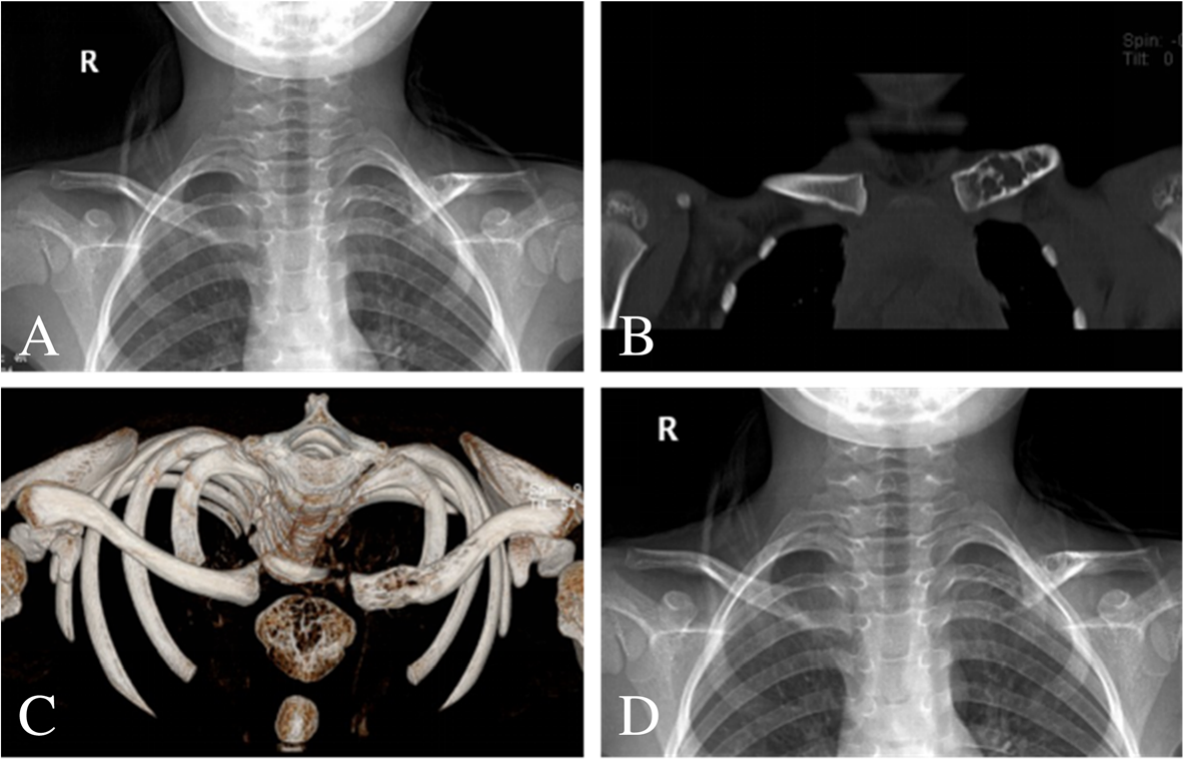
A 5-year-old male with left, medial, and clavicular simple bone cysts. a Preoperative plain X-ray. b, c CT shows an obvious tumor destruction on the medial left clavicle. d Postoperative X-ray
